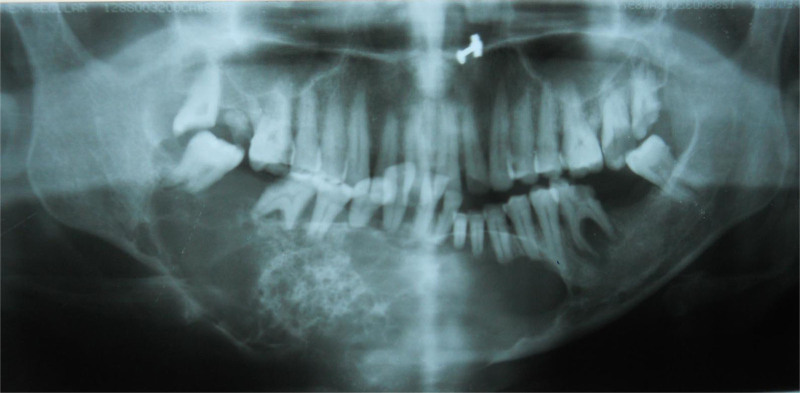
OPG showing a multilocular radiolucent lesion with “tooth floating in air” appearance. OPG = orthopantomogram.
